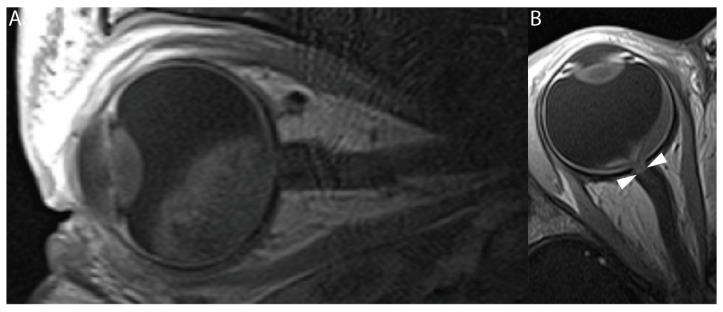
Baseline and posttreatment T1-weighted contrast-enhanced MRI of a unilateral retinoblastoma patient. (A) Sagittal contrast-enhanced T1-weighted MR image of a 13-month-old patient with unilateral retinoblastoma. This pretreatment image does not show an increased enhancement of the distal optic nerve. (B) Axial contrast-enhanced T1-weighted MR image of the same patient performed after the last SIAC course (21 months of age). The patient received a total of six cycles of combined 24 mg melphalan (4 mg per cycle) and 6 mg of topotecan (1 mg per cycle). Increased contrast enhancement can be seen in the most distal part of the optic nerve (white arrowheads). After enucleation, tumor invasion was ruled out, suggesting that the increased enhancement of the optic nerve was most likely caused by an SIAC-induced inflammatory event.
